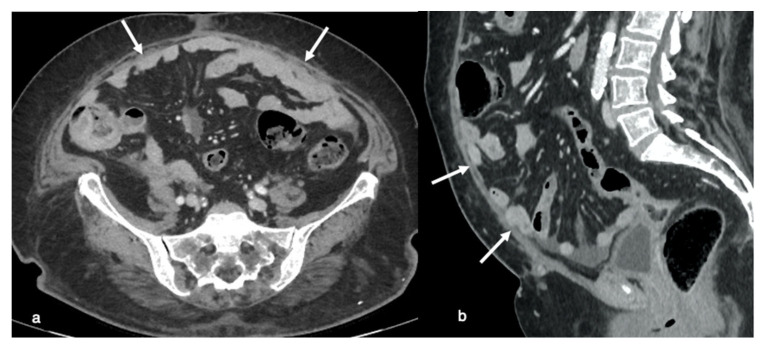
Relative contraindications to PIPAC or laparoscopic approaches. Axial (a) and sagittal MPR (b) contrast-enhanced CT-images show a focal obscuration of pro-peritoneal fat associated with closely adherent omentum and small bowel loops (arrows), indicative of anterior entero-parietal adhesions.
